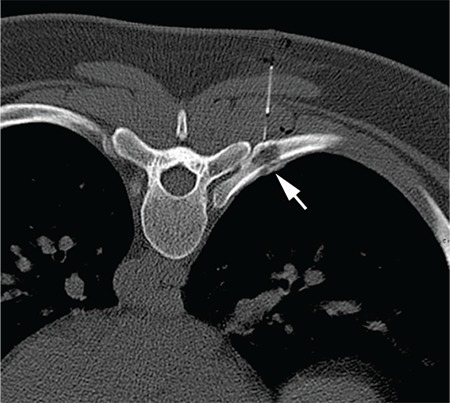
CT guided hookwire localization and injection of blue dye priorto an excision biopsy of the lesion (solid white arrow)
